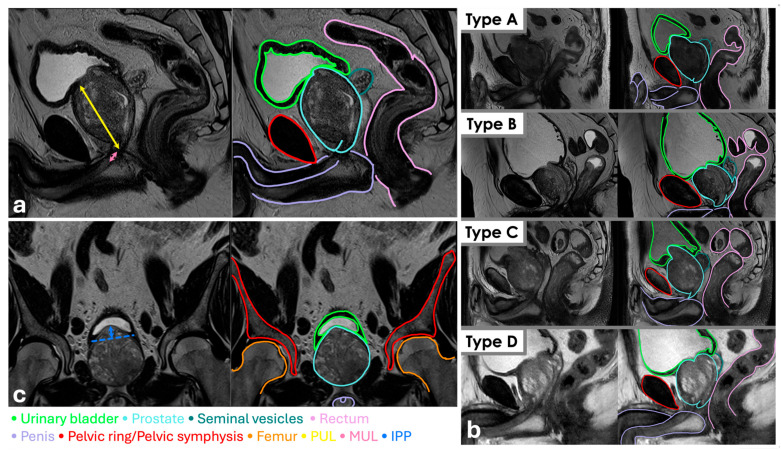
Multiparametric magnetic resonance imaging (MRI) of the male pelvis with anatomical annotation and morphological Lee Types classification. (a) Sagittal views; (b) Examples of different sagittal morphological variants of the urethro–prostatic junction and pelvic relationships (Types A–D); (c) Coronal views.
